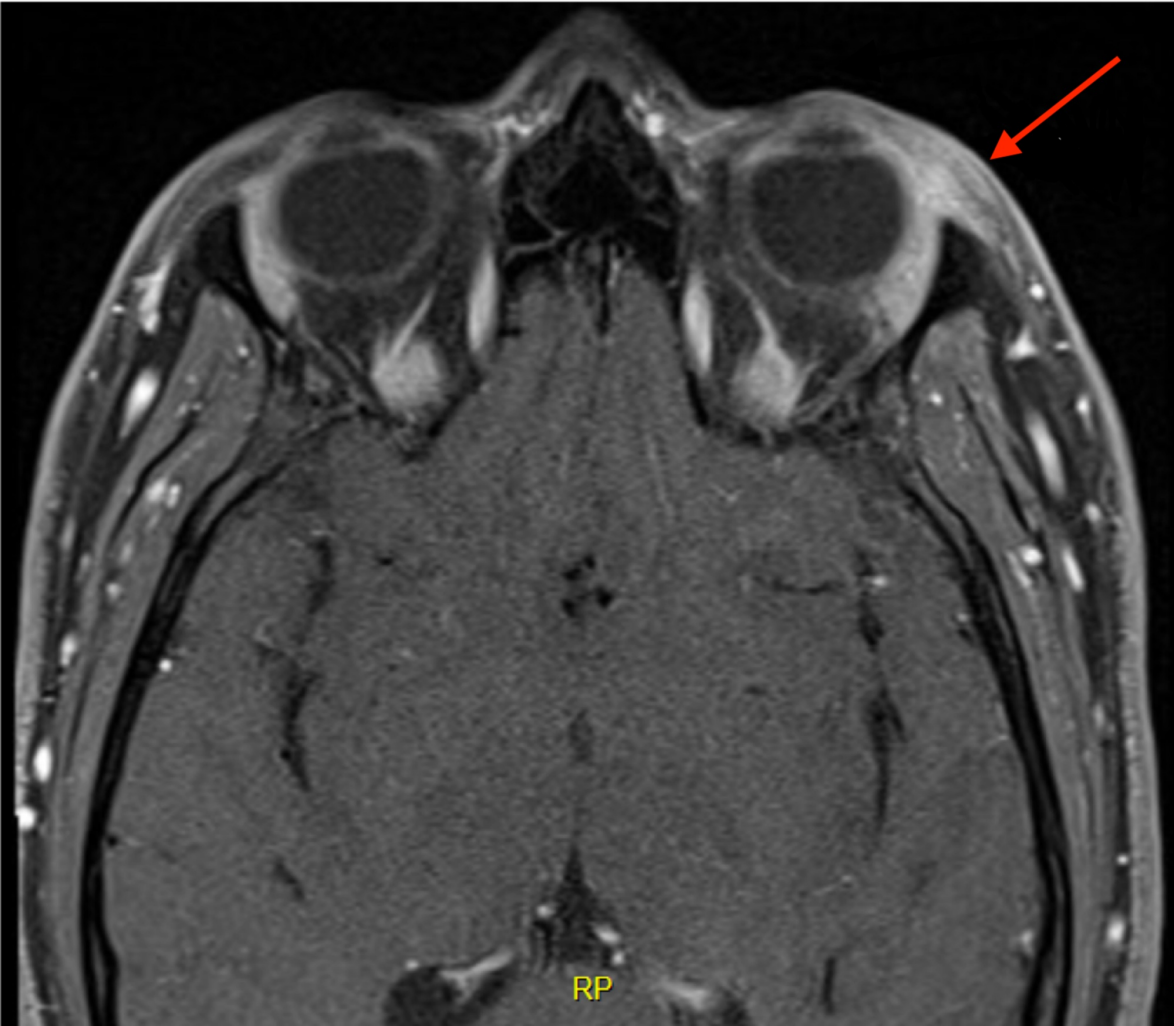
Orbital MRI showing increased enhancement of the left lateral rectus muscle (arrow).
